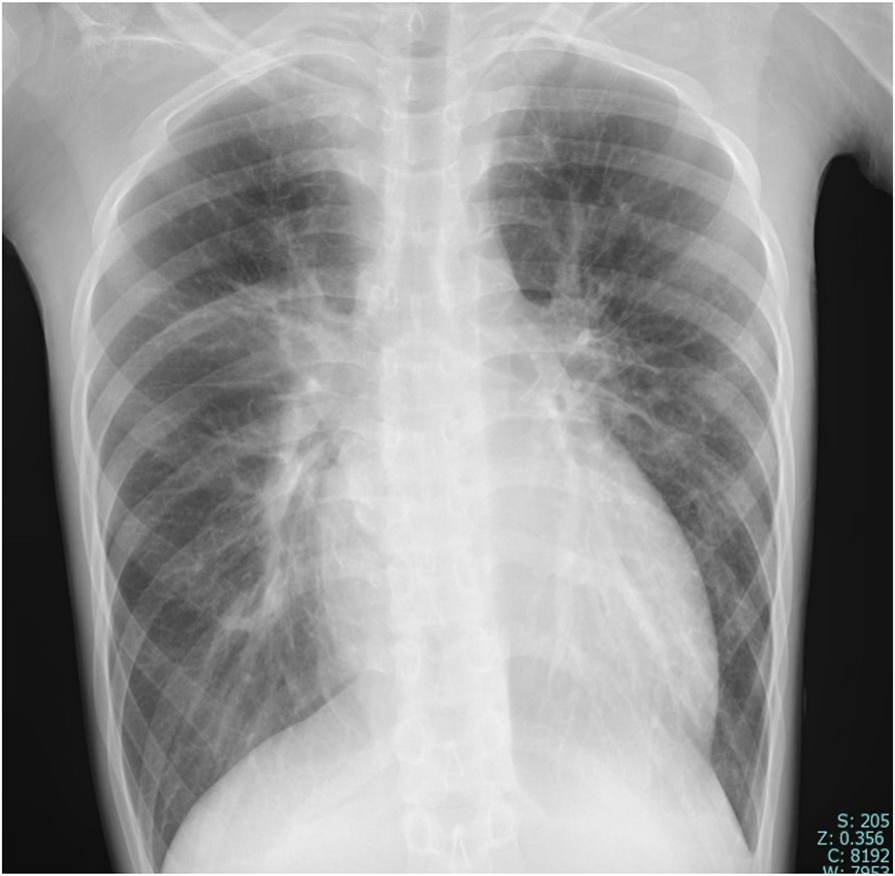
Chest X-ray at admission. The X-ray shows the enlargement of a cardiac shadow with a cardiothoracic ratio of 0.62, accompanied by severe pulmonary congestion
